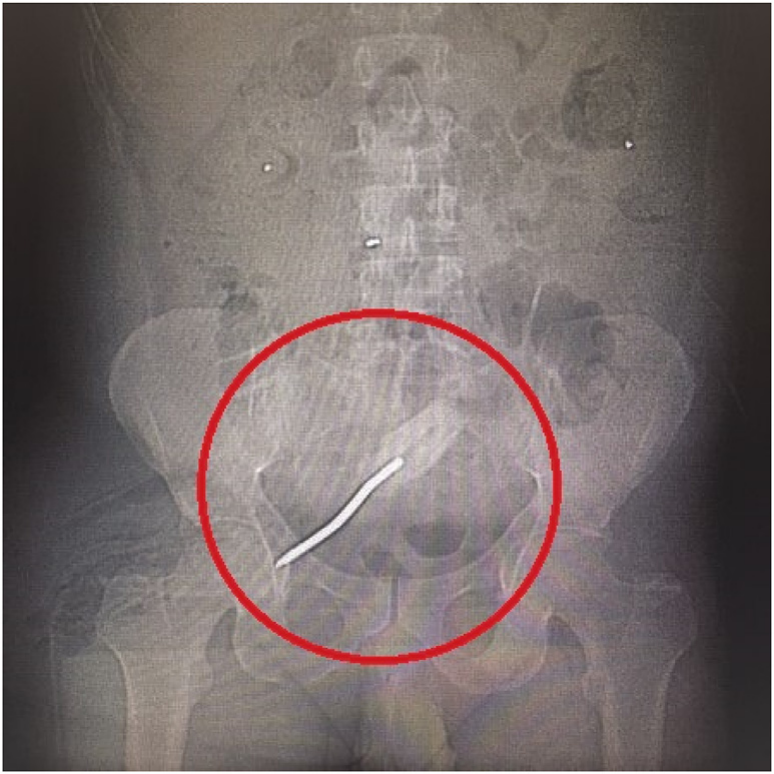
CT scout image with the foreign body identified as a screwdriver, circled in red.
